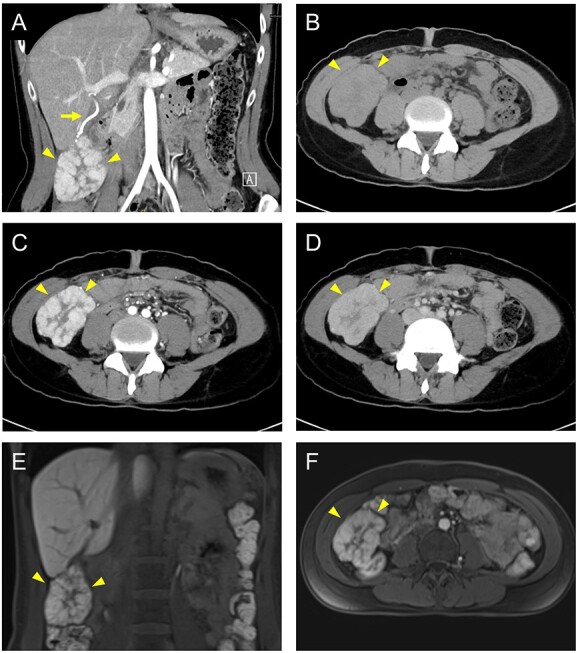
Findings of dynamic contrast-enhanced CT (A–D) and dynamic magnetic resonance imaging (E and F). (A) Coronal image demonstrates an exophytic hyperdense mass (arrowheads) and its feeder artery (arrow). (B) Native examination shows an isodense mass (arrowheads). (C) The multilobular mass is hyperdense and enhanced with a central stellate scar at the arterial phase (arrowheads). (D) The mass is still enhanced at the portal venous phase (arrowheads). On coronal (E) and axial (F) fat-saturated contrast-enhanced T1-weighted images, the mass demonstrates strong uptake of contrast agent at the early phase (arrowheads).
